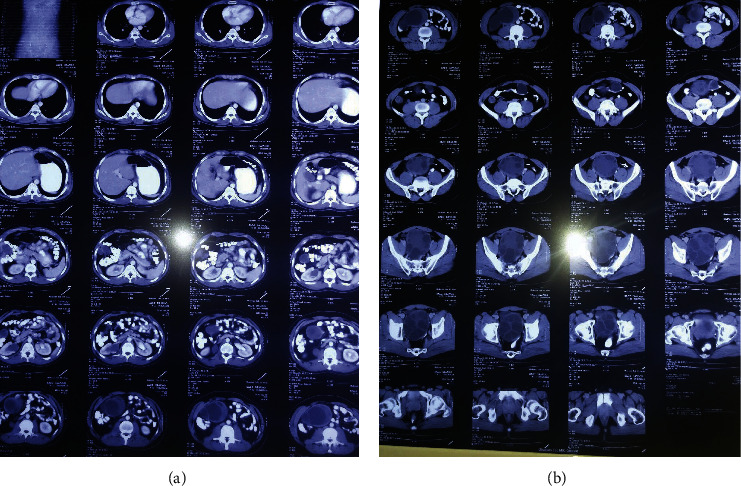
CT showing no involvement of the liver (a). CT showing a pelvic hydatid cyst with hydroureteronephrosis (b).
